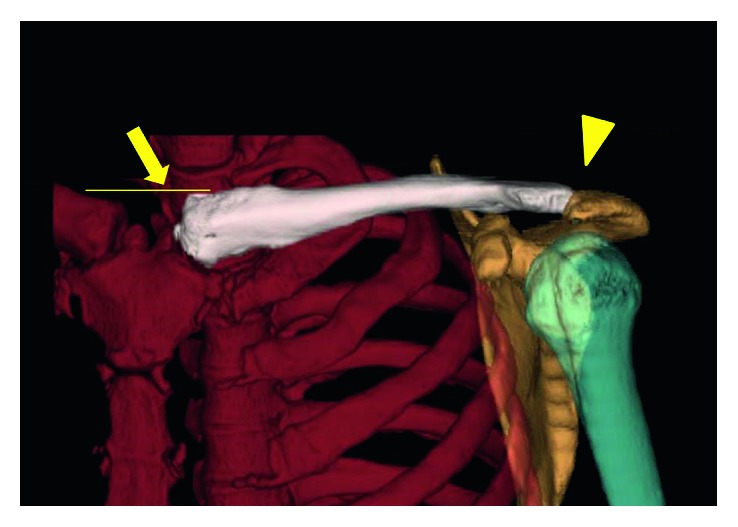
Case 2: a three-dimensional reconstructed CT scan of the SCJ at 12 months' follow-up shows a reduced ACJ (arrowhead) and SCJ (arrow) with remaining 2.5 mm superior displacement of the clavicle (line).
